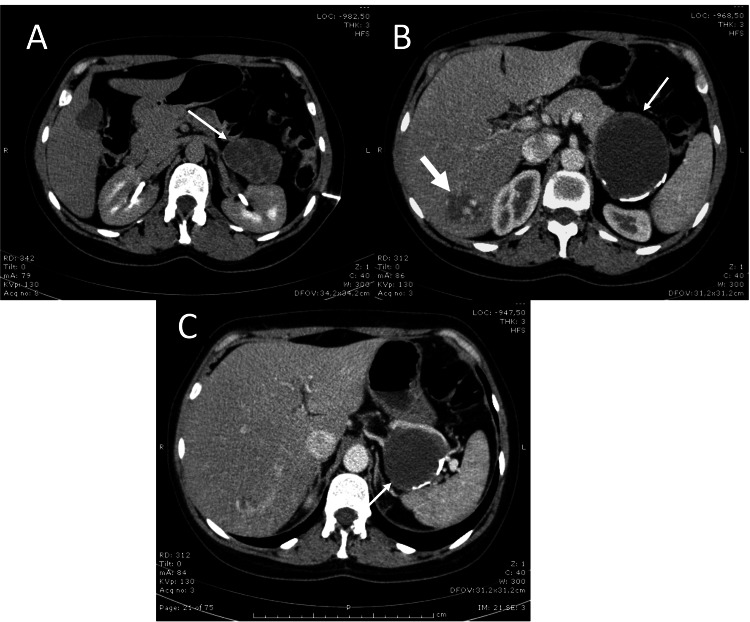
CT images of the clinical case. A lesion with solid and liquid components, delimited by a partially calcified capsule (narrow arrows), localized on the pancreatic tail; angiomatous lesion of the right hepatic lobe is also evident (bold arrow) (A, B, and C).
